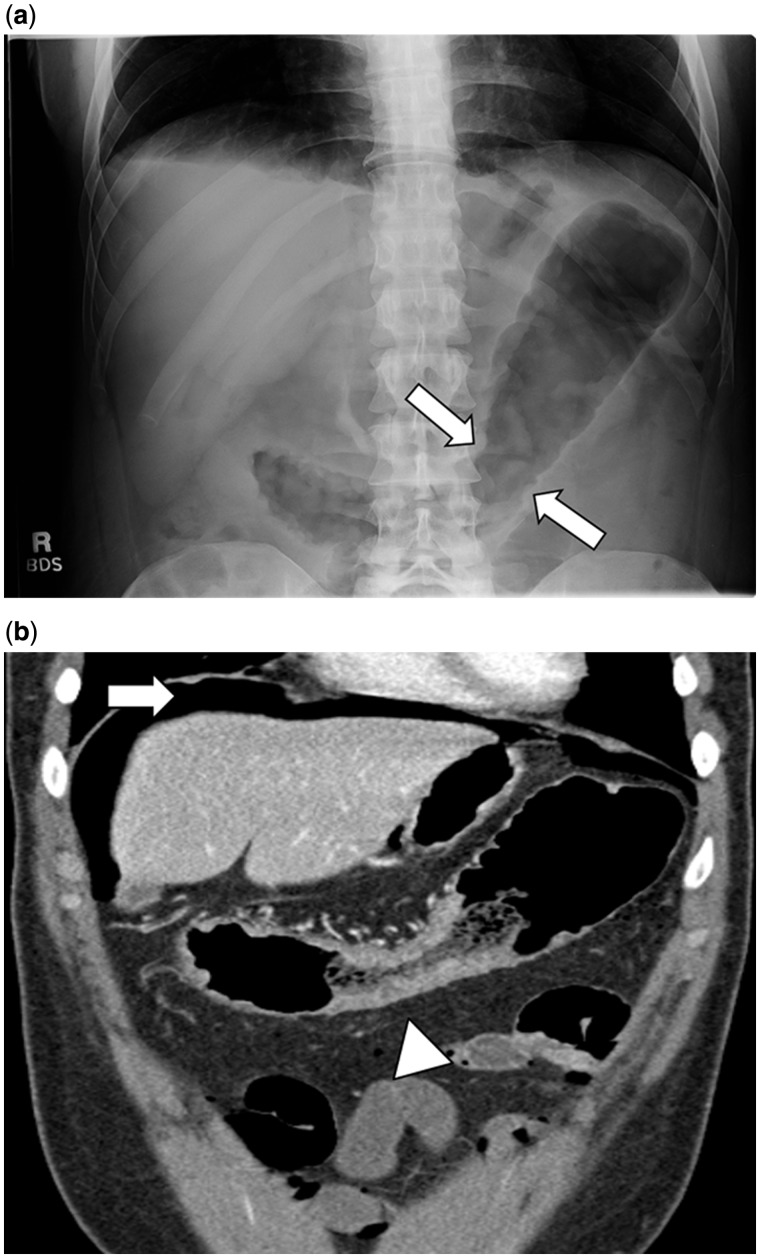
A 32-year-old female patient with ulcerative colitis presenting with abdominal pain and bloody diarrhea. 1a: Abdominal X-ray depicting areas of thumbprinting (white arrow) suggestive of colonic bowel wall edema. 1b: Computed tomography demonstrating free air (white arrow) and colon wall thickening (white arrowhead).
